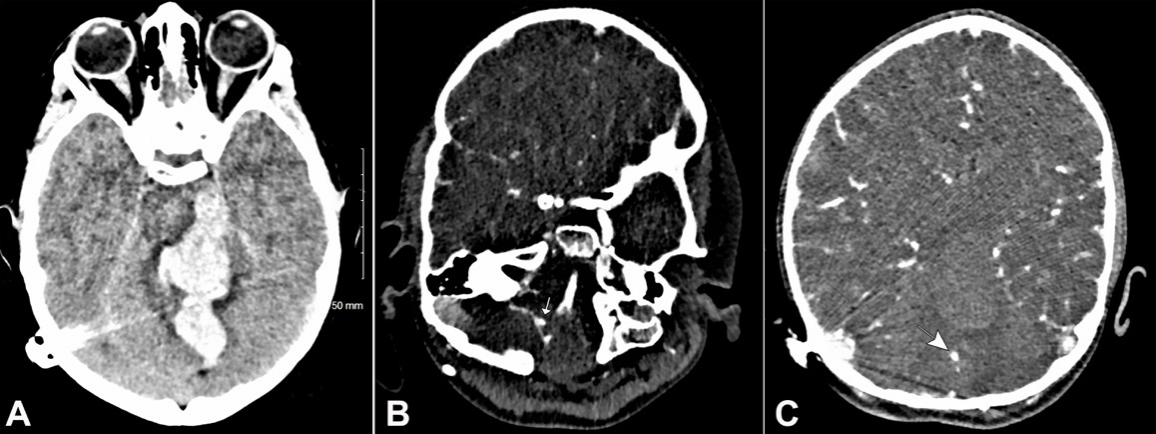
CT brain on the second presentation. A – Intracerebral hemorrhage in the cerebellar vermis with extension into the distorted fourth ventricle, the third and lateral ventricles, the quadrilateral cistern, and the left cerebral peduncle with adjacent vasogenic edema; B – CTA showing aneurysm of the right superior cerebellar artery (arrow); C – Proximal aneurysm of right PICA (arrow).
